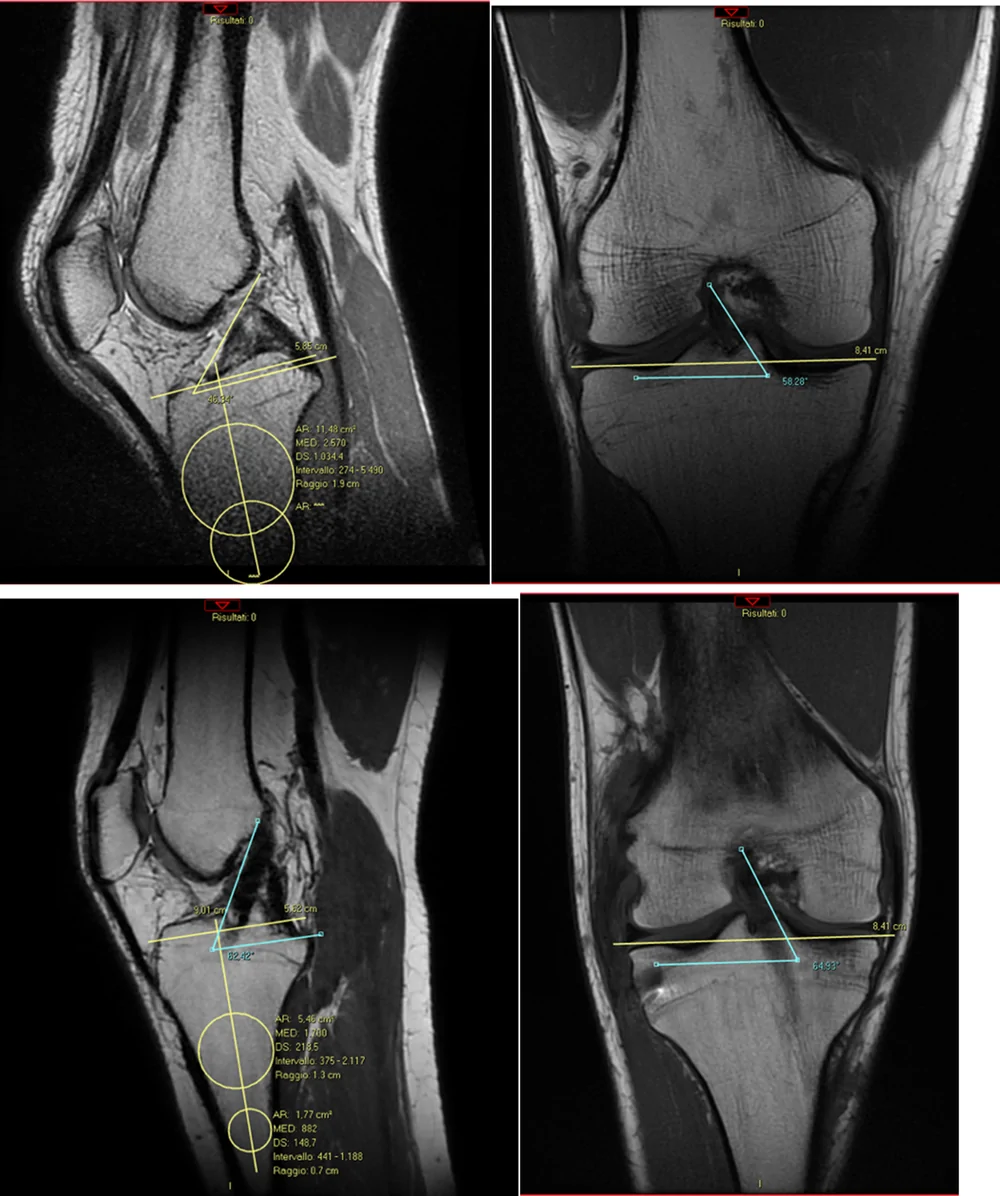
MRI assessment of graft orientation. (a) Sagittal view of the native ACL demonstrating the sagittal inclination angle (SIA) of 46.3°. (b) Coronal view of the native ACL demonstrating the coronal inclination angle (CIA) of 58.3°. (c) Postoperative sagittal MRI following OTT plus LET ACL reconstruction demonstrating the graft SIA of 62.4°. (d) Postoperative coronal MRI demonstrating the graft CIA of 64.9°. ACL, anterior cruciate ligament; LET, lateral extra‐articular tenodesis; MRI, magnetic resonance imaging; OTT, over‐the‐top.
